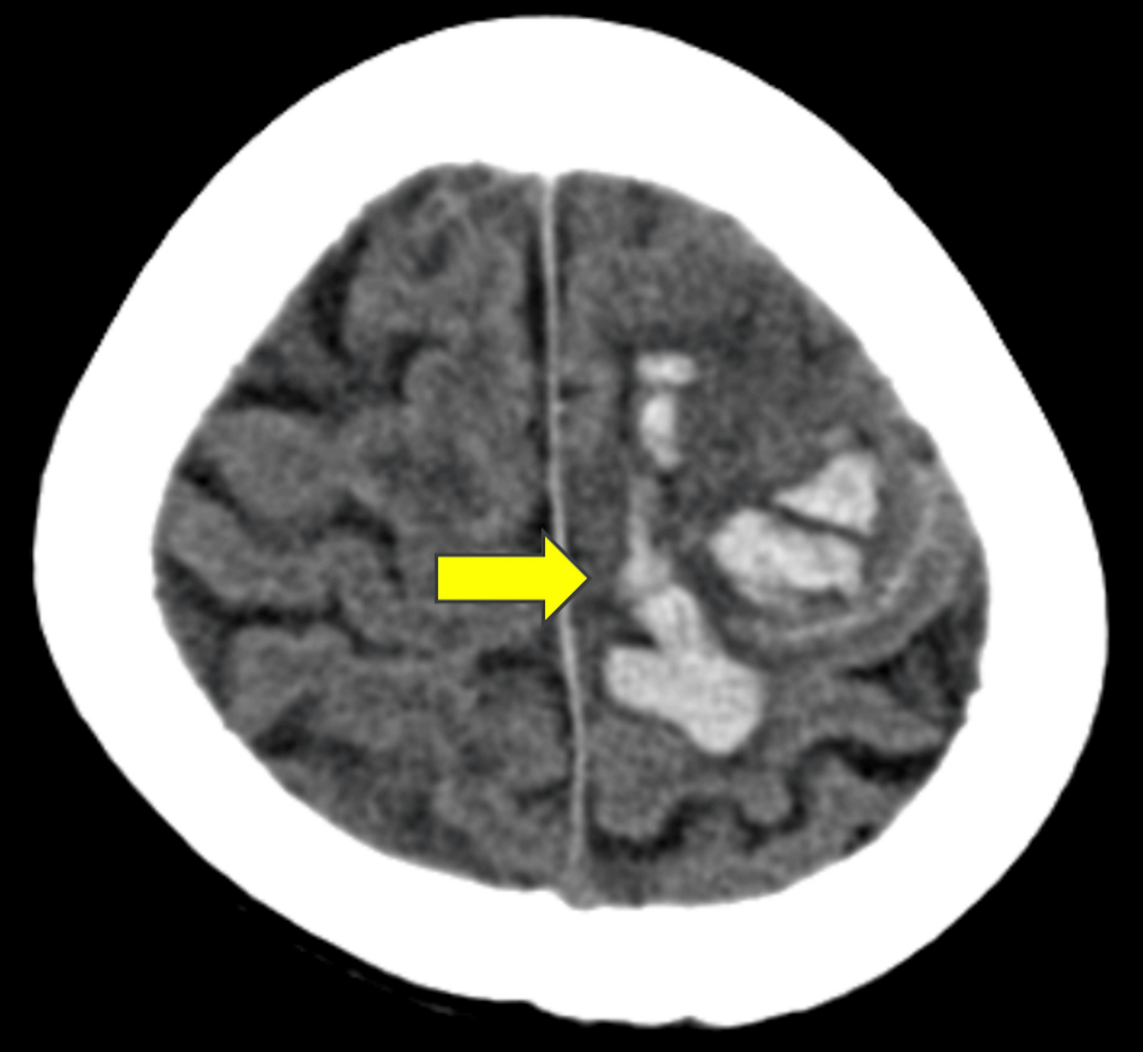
The patient's head CT on admission revealed subcortical hemorrhage in the left frontal to parietal lobes. CT: computed tomography
